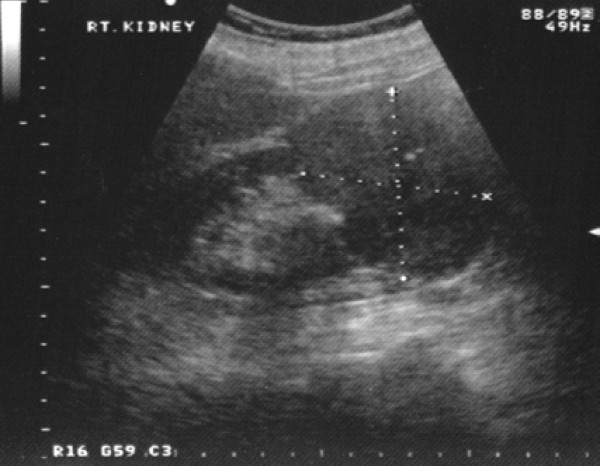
Ultrasound examination reveals a large mass on the upper pole of the patients' right kidney.
